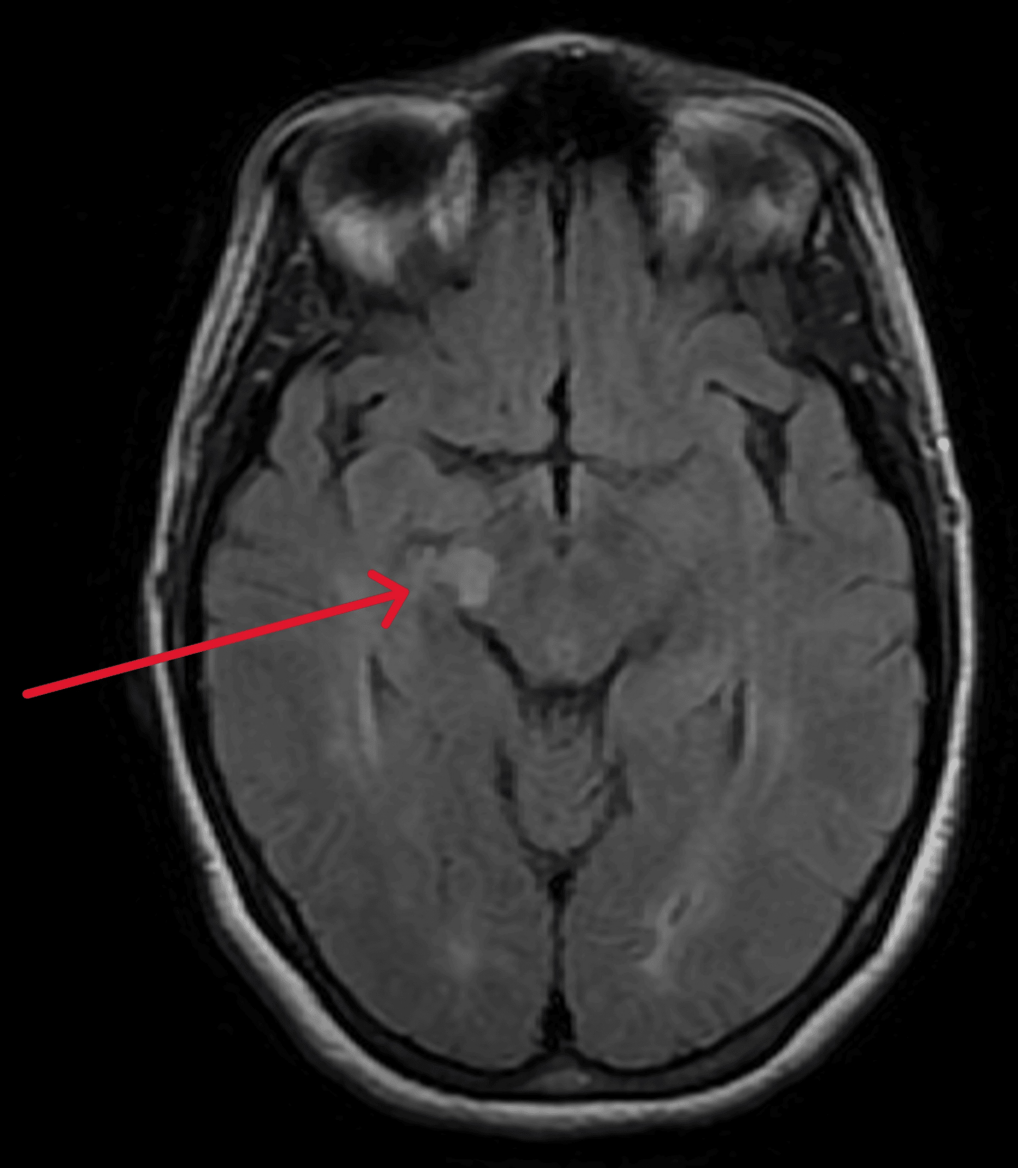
Axial T2-FLAIR MRI demonstrating a new hyperintense lesion in the lateral part of the right cerebral peduncle. The arrow indicates the demyelinating lesion located in the lateral cerebral peduncle on MRI. FLAIR: fluid-attenuated inversion recovery
